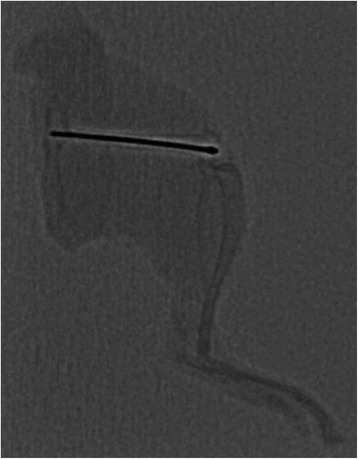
Lateral X-ray of the intramedullary compressed femur ten weeks postoperatively
